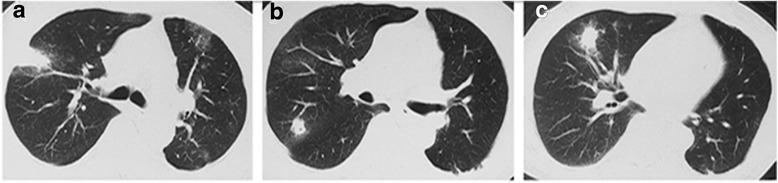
Pulmonary CT scan revealing multiple nodules in bilateral lung, including right upper lobe (a and b) and right middle lobe (c), which proved recurrence of lung cancer later by biopsy. (2016-3-21)
